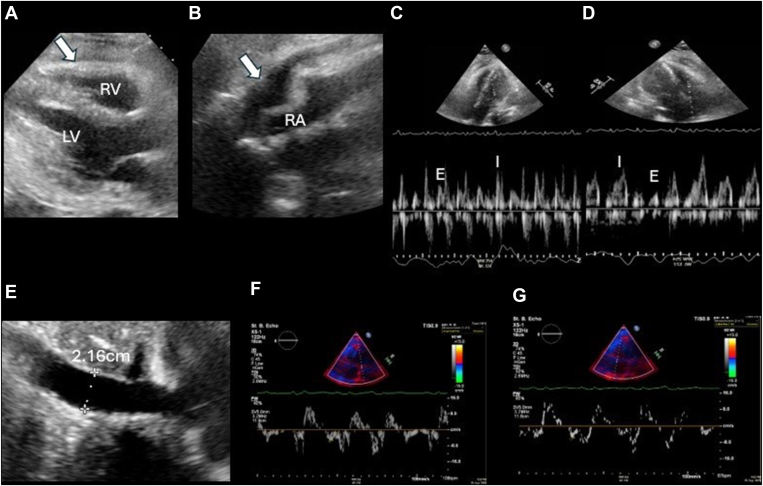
Echocardiographic findings of effusive-constrictive pericarditis. (A) Parasternal long-axis (PLAX) view revealing a thick pericardium alongside the right ventricle (RV; arrow). (B) Subcostal view with pericardial effusion causing right atrial (RA) diastolic collapse (arrow). (C, D) Mitral valve and tricuspid valve respirophasic inflow variation. (E) Dilated inferior vena cava measuring 2.16 cm. (F, G) Annulus reversus with the septal e’ velocity (6.4 cm/s) being higher than the lateral e’ (5.8 cm/s). E, expiration; I, inspiration; LV, left ventricle.
